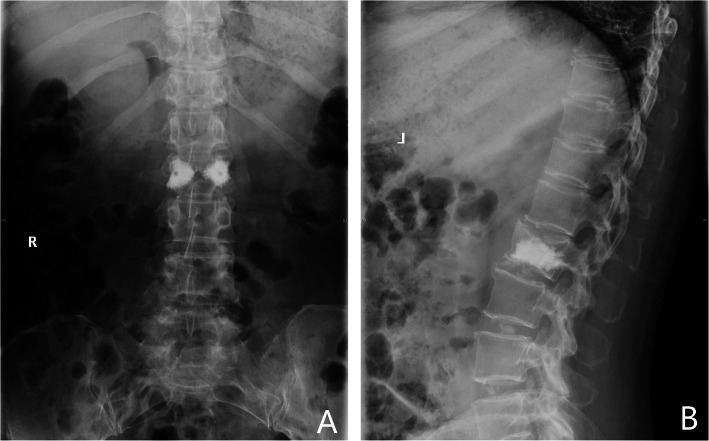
Anteroposterior and sagittal X-ray of lumbar spine demonstrate PMMA filled the L2 inferior endplate and vertebral body around SN at 1 month
